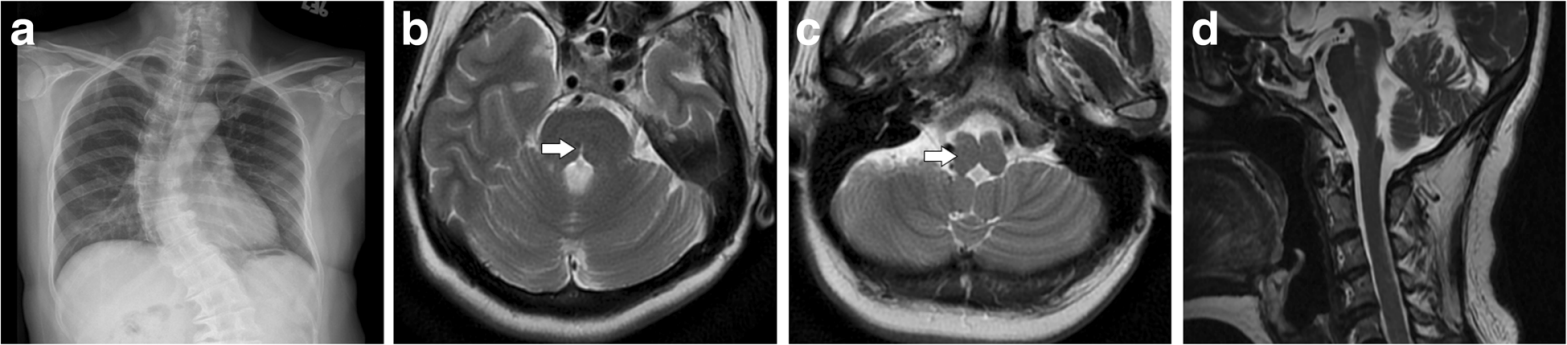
Main conventional imaging findings of this patient with horizontal gaze palsy with progressive scoliosis. a Chest X-ray showing S-shaped scoliosis of the thoracolumbar spine. b Axial T2-weighted brain magnetic resonance imaging showing a midline cleavage at the dorsal aspect of the pons (split pons sign) (arrow). c Butterfly configuration of the medulla (arrow). d Atrophic change of the pons in sagittal view
